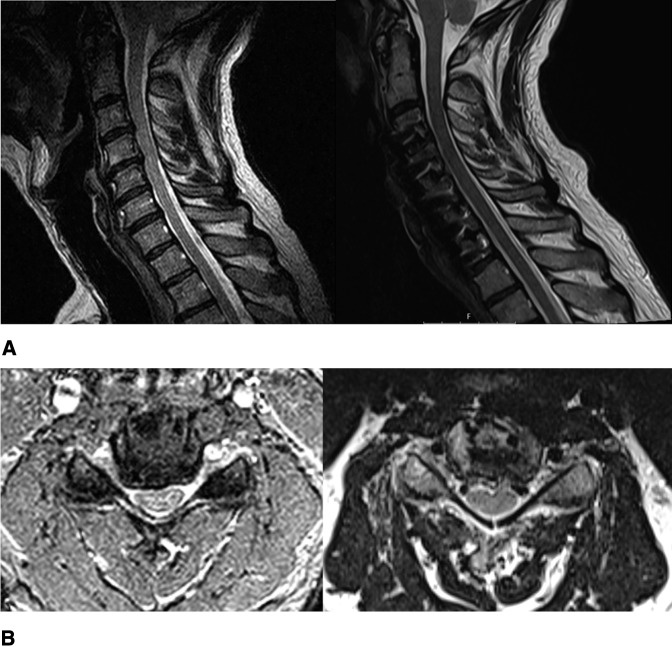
MRI scan of the cervical spine showing (A) sagittal view: (left) preoperative image demonstrating central spinal canal stenosis at C3-4 and C4-5 without obvious cord signal change, (right) postoperative. B, Axial view of the C4-5 level: (left) preoperative image demonstrating right-side dominant foraminal stenosis and central spinal canal stenosis with cord compression, (right) postoperative.
